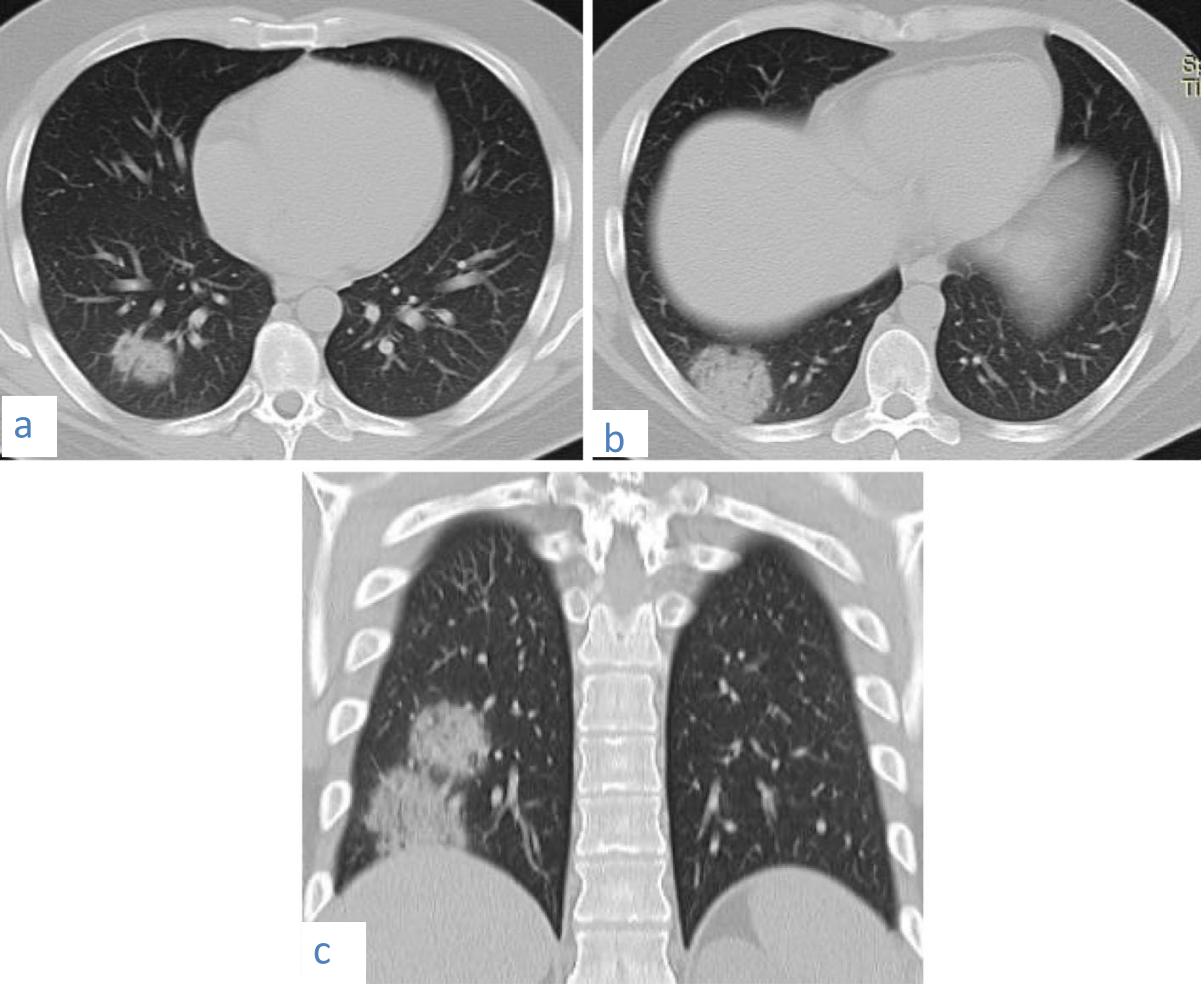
A 27-year-old male patient with COVID-19. CT chest axial images (a) and (b) and coronal image (c) show two subpleural masses showing “vacuolar” sign
